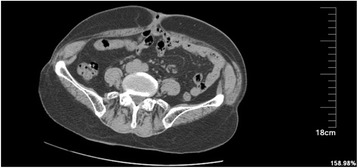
A CT scan showing a loop of intestine lumen was entrapped in the anterior abdominal wall
